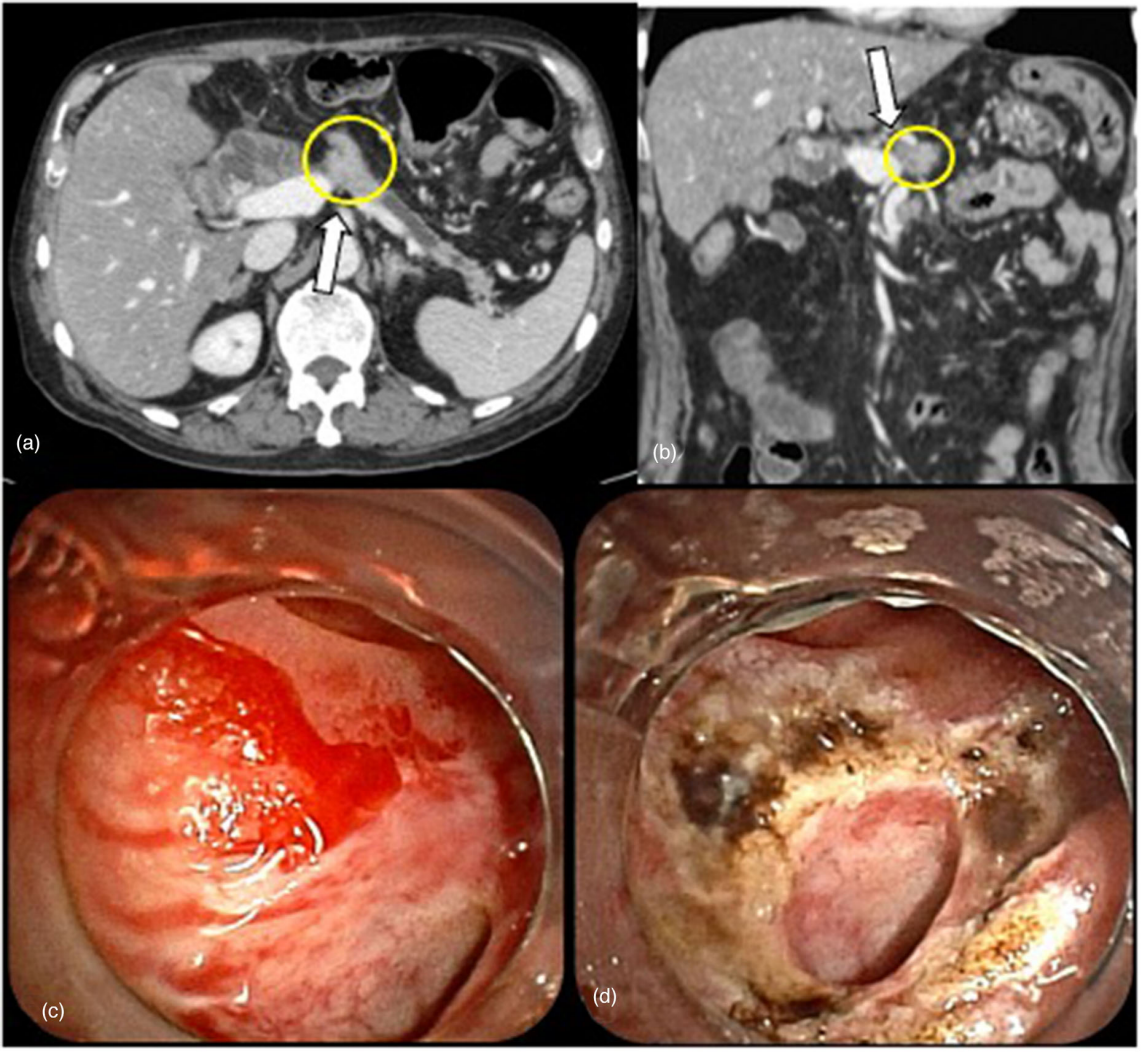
In case 2, contrast‐enhanced computed tomography showed portal vein stenosis due to recurrent tumor ((a) axial view, (b) coronal view). Endoscopic image shows the angiodysplasia‐like lesions around the hepaticojejunal anastomosis (c), and we employed argon plasma coagulation (d). White arrow: portal vein stenosis, yellow circle: recurrent tumor
